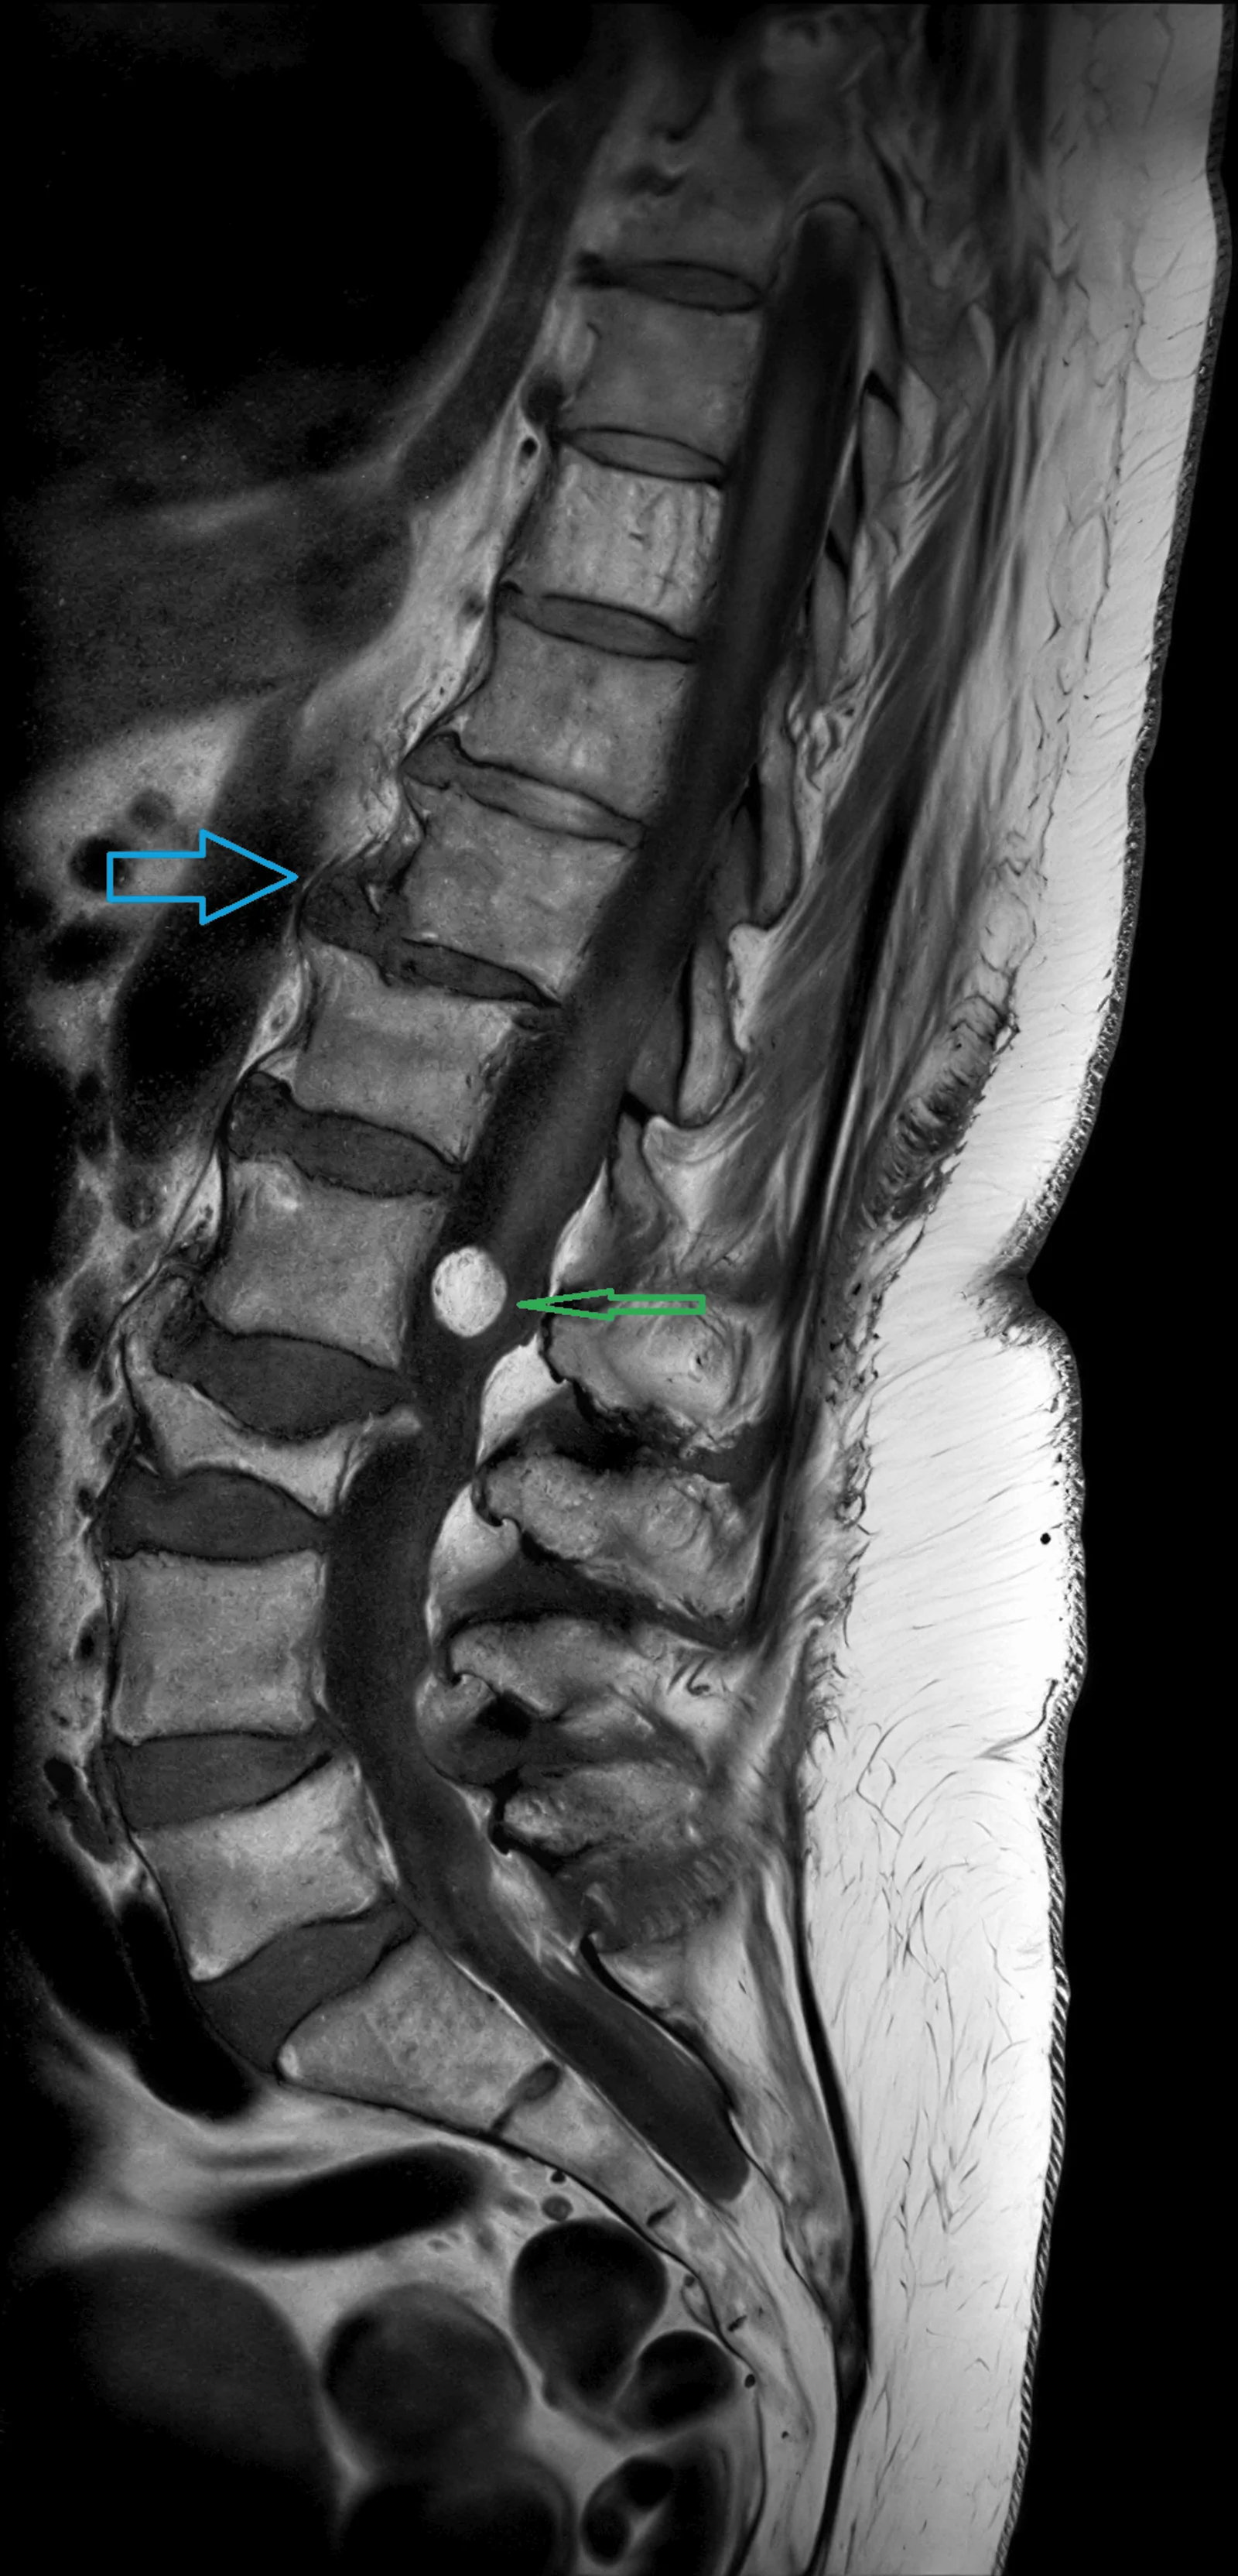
Initial MRI of lumbosacral spine without contrast T12 - L1 disc protrusion indenting the thecal sac indicated with blue arrow, and incidental intradural lipoma of the conus indicated with green arrow
